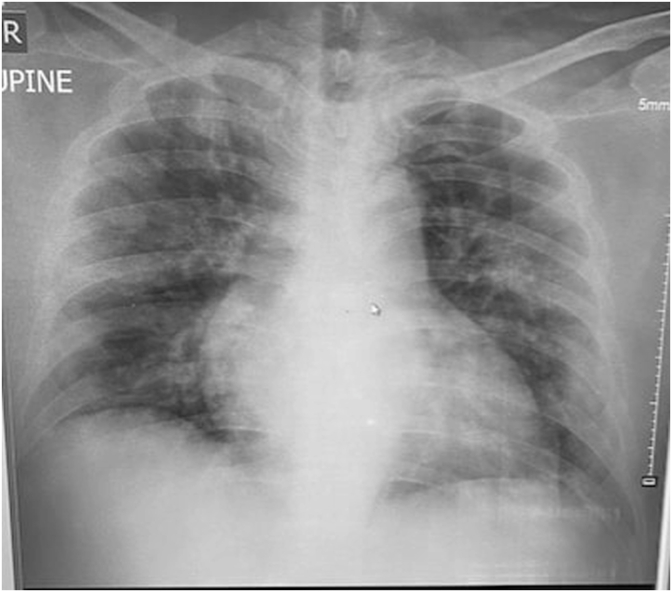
Plain chest X-ray presented GGOs in the periphery of the left lung and consolidation in the bilateral parahilar and paracardial regions of the lung.
